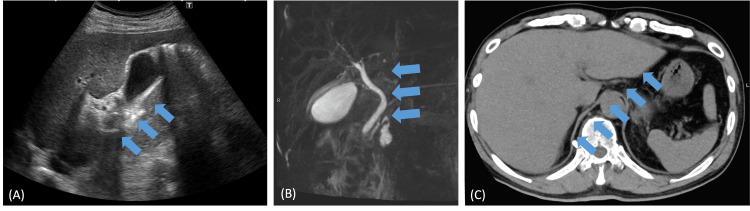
Imaging studies of the hepatobiliary system (A) Ultrasonography, (B) magnetic resonance cholangiopancreatography, and (C) non-enhanced computed tomography. No morphological abnormality was found in the hepatobiliary system (arrows).
